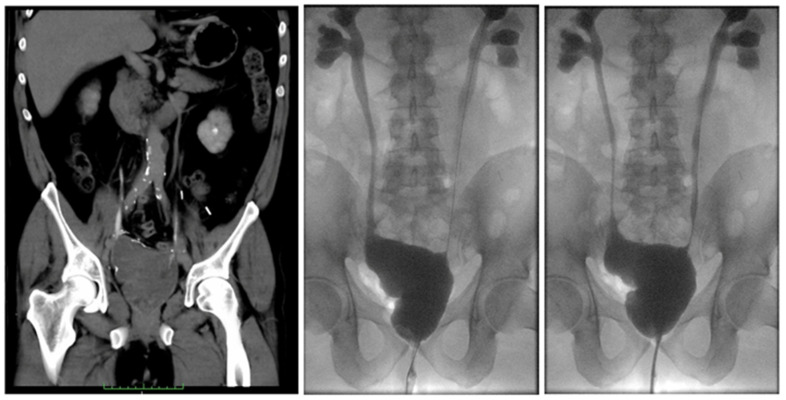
Outcome after open surgical correction of the stenosis in our hospital. (Left) CT-urography. (Center and right) cystography and bilateral retrograde ureteropylography images at different time points. Green scale bar: cms.
